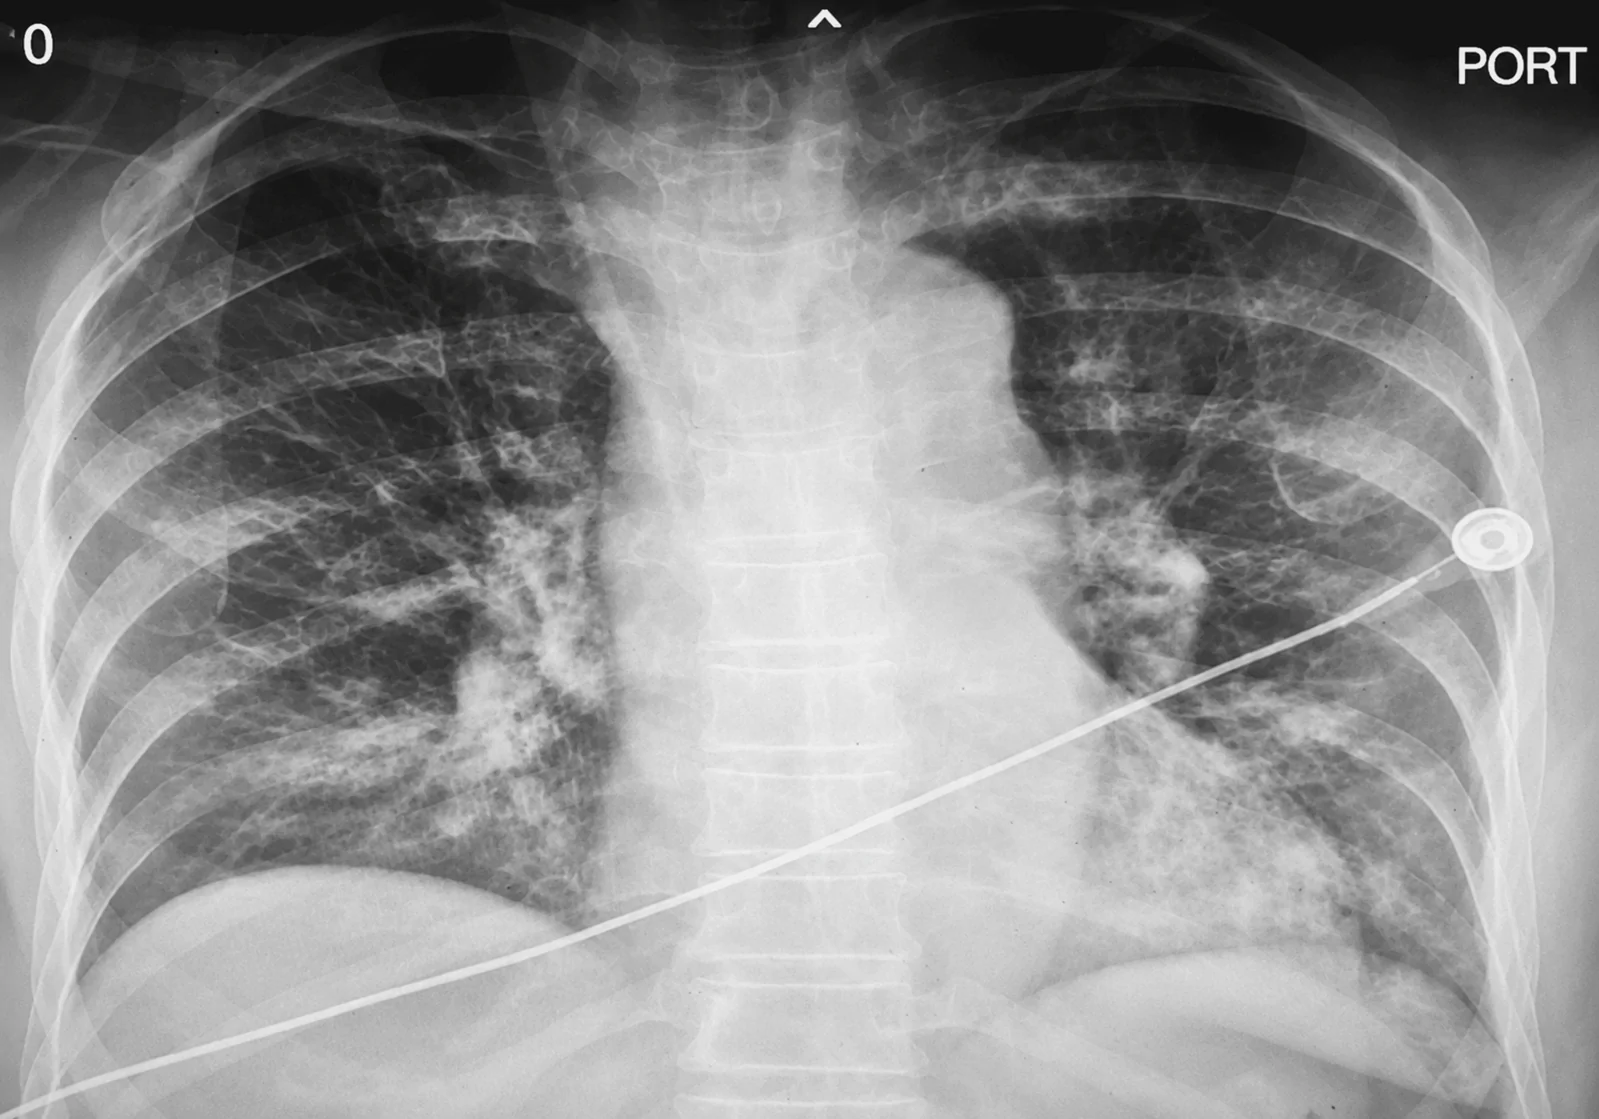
Chest radiograph on the day of admission (D1) showing no pleural effusion or radiographic evidence of capillary leak.
